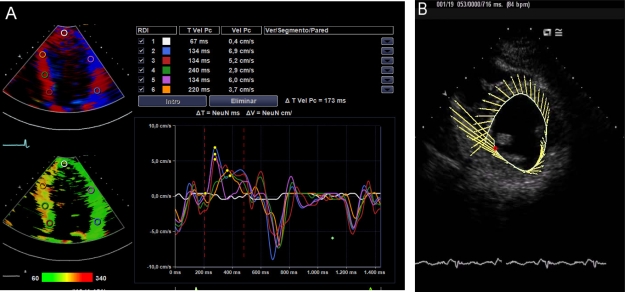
New echocardiographic techniques. A) Advanced DTI, B) Speckle tracking.
